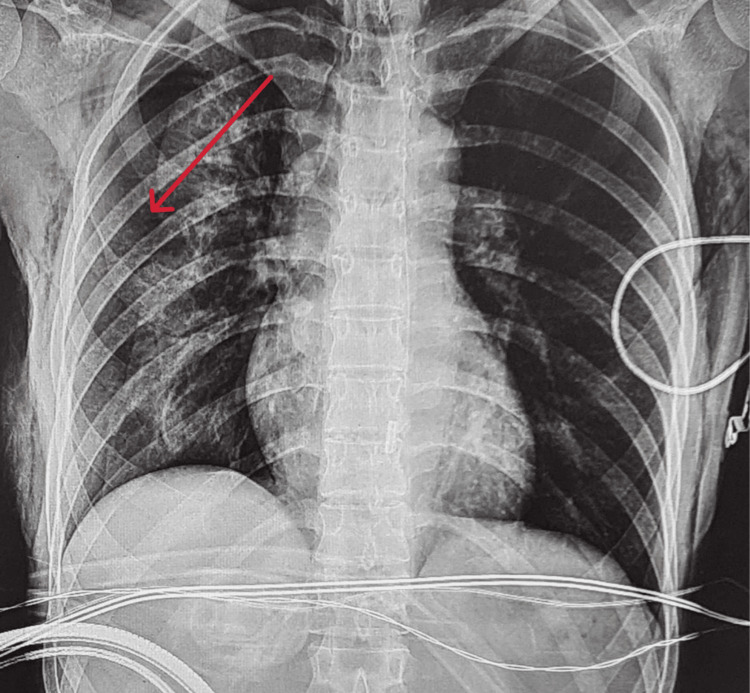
Intraoperative X-ray (AP view in the supine position) confirming the presence of right-sided pneumothorax
